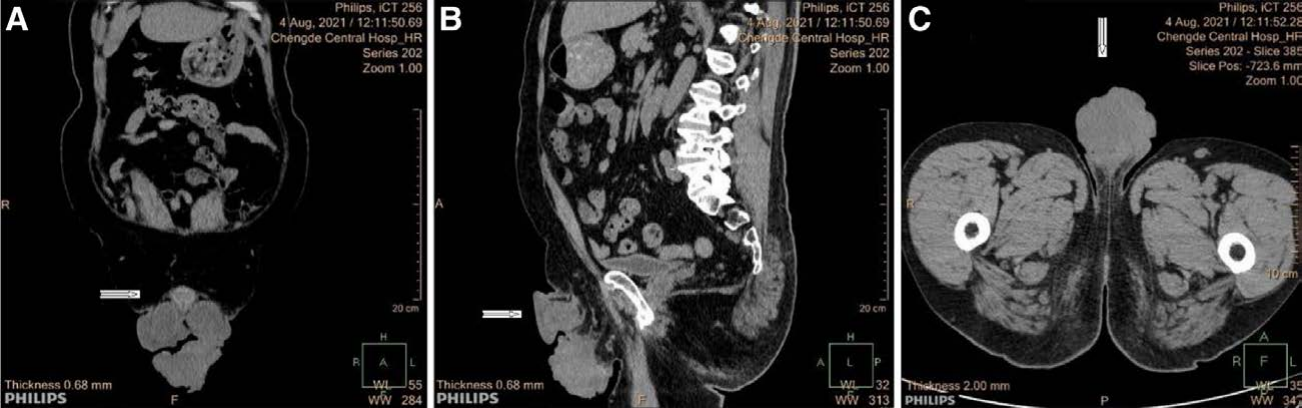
CT of the tumor. (A) In the coronal plane, (B) sagittal plane, and (C) horizontal plane, large tumor tissue and lymph node metastasis can be seen. CT = computed tomography.
